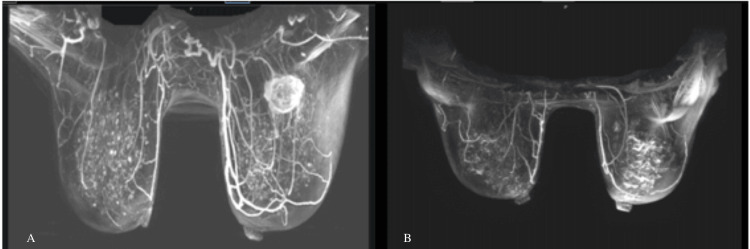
Breast MRI Breast MRIs before treatment (A) and after treatment (B). A: the heterogeneous mass in the left breast along the posterior breast tissue; B: a lack of mass.
